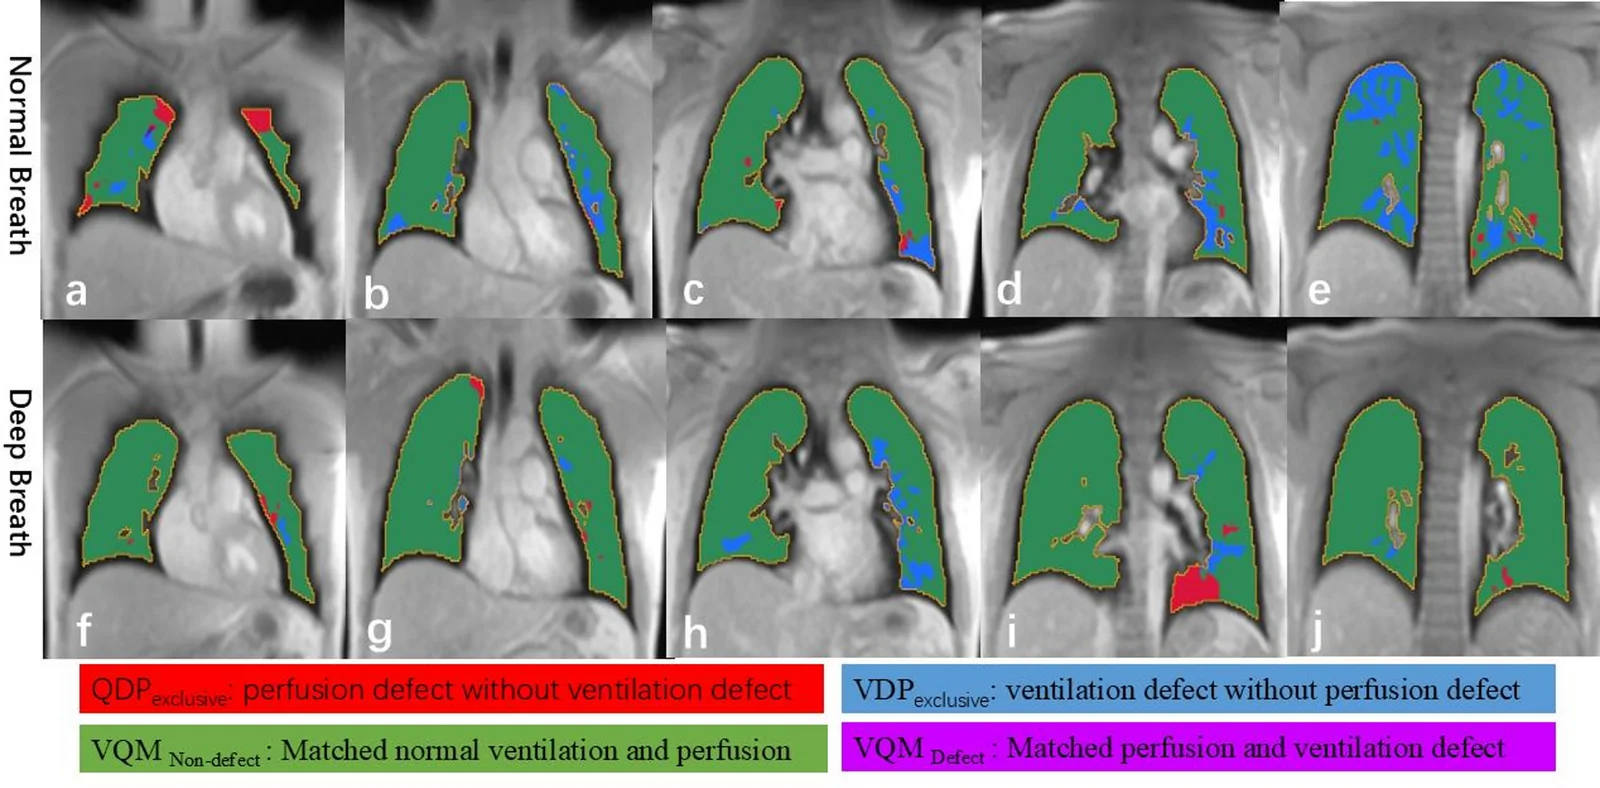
Whole-lung ventilation and perfusion maps acquired using PREFUL-MRI in a healthy 45-year-old man during normal breathing (Row 1, panels a–e, anterior to posterior) and deep-slow breathing (Row 2, panels f–j, anterior to posterior). Color coding: red, QDP_exclusive_ (perfusion defect without ventilation defect); blue, VDP_exclusive_ (ventilation defect without perfusion defect); green, VQM_non−defect_ (matched normal ventilation and perfusion); purple, VQM_defect_ (matched perfusion and ventilation defect). Abbreviations: PREFUL = Phase-resolved Functional Lung
